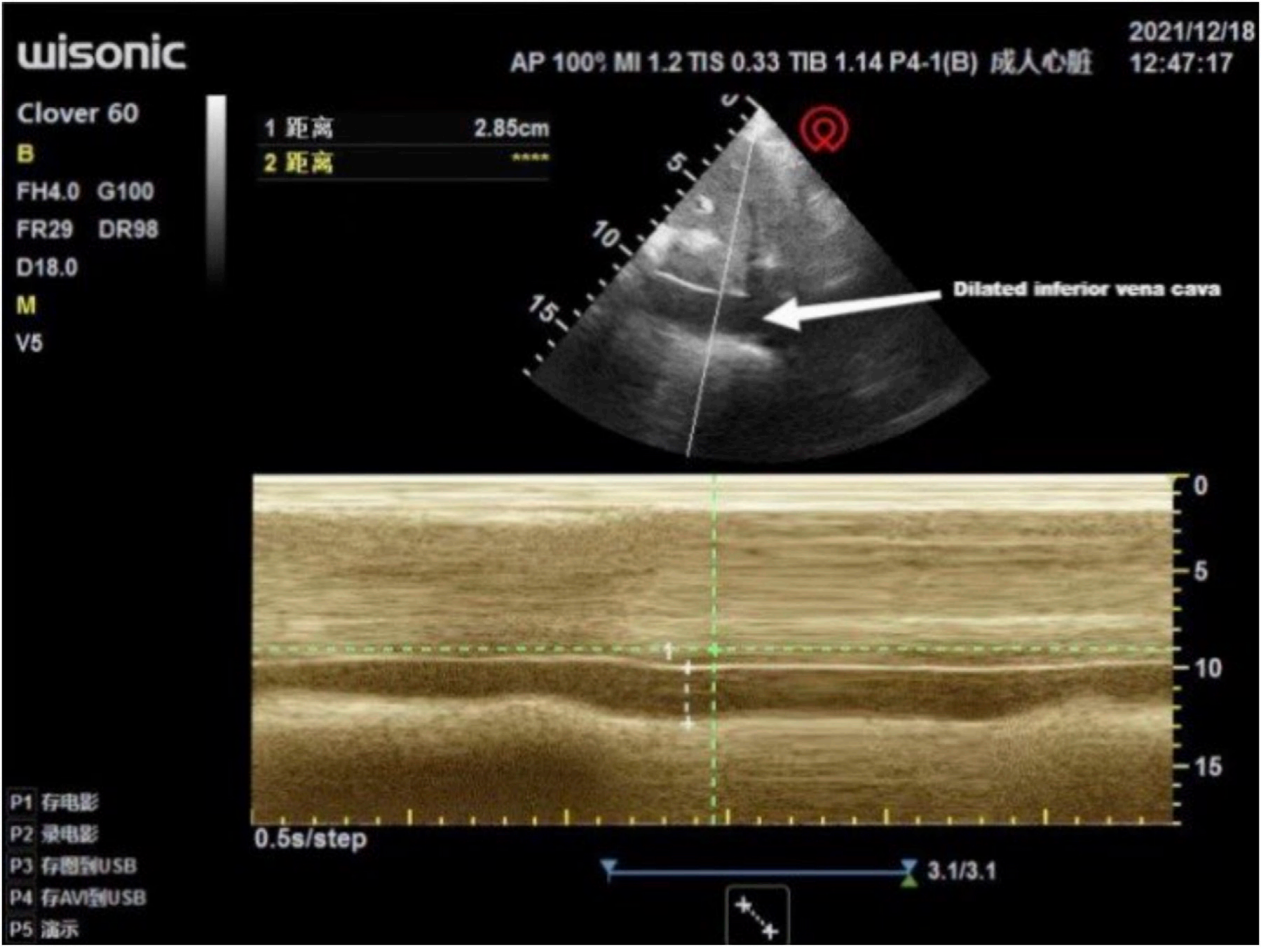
Transthoracic echocardiography showed a dilated inferior vena cava: 2.85 cm in diameter.
